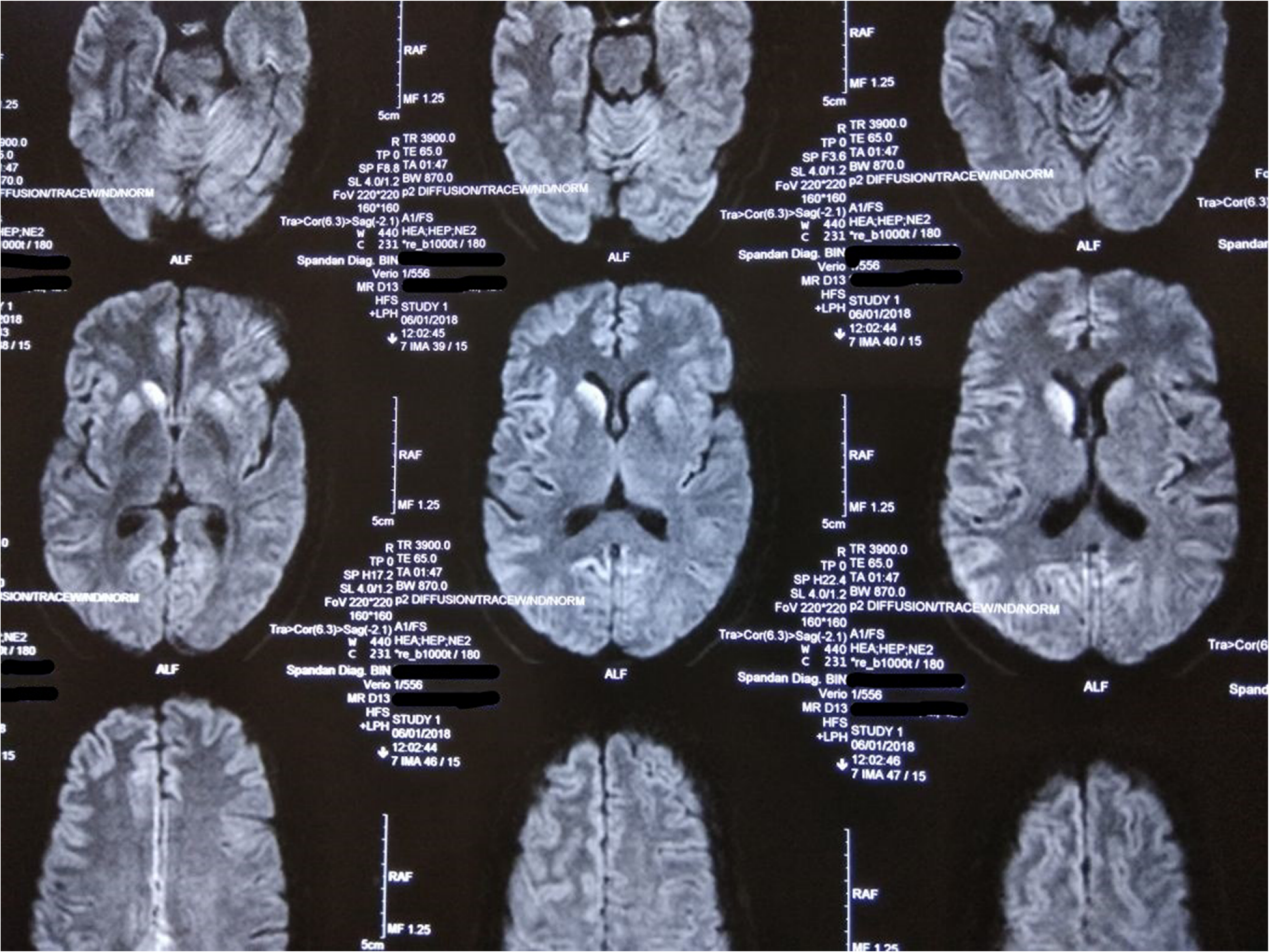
Magnetic resonance imaging brain diffusion-weighted sequence revealed hyperintensity in bilateral caudate nucleus and putamen. Diffusion restriction is also observed in bilateral parieto-occipital and insular cortex (more on right side) resembling typical cortical ribbon pattern of sporadic Creutzfeldt–Jakob disease
